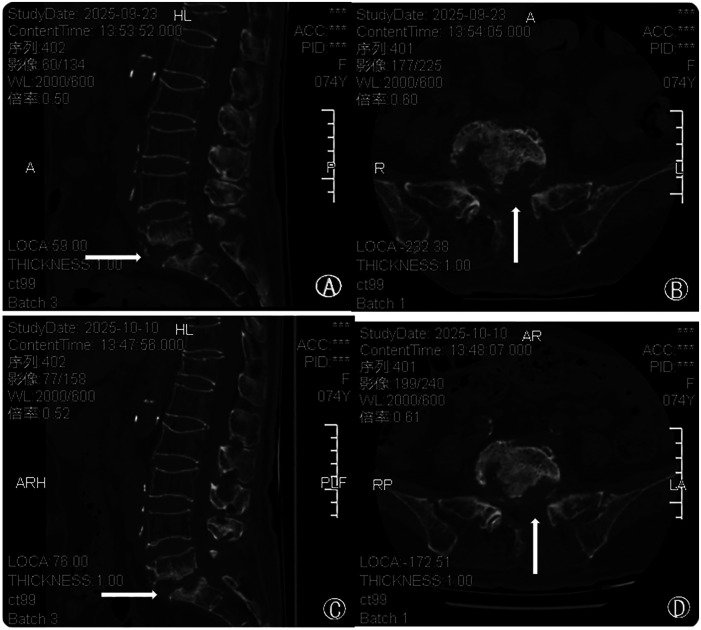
CT scan showed improvement in L5 and S1 vertebral bone defects compared to before.
